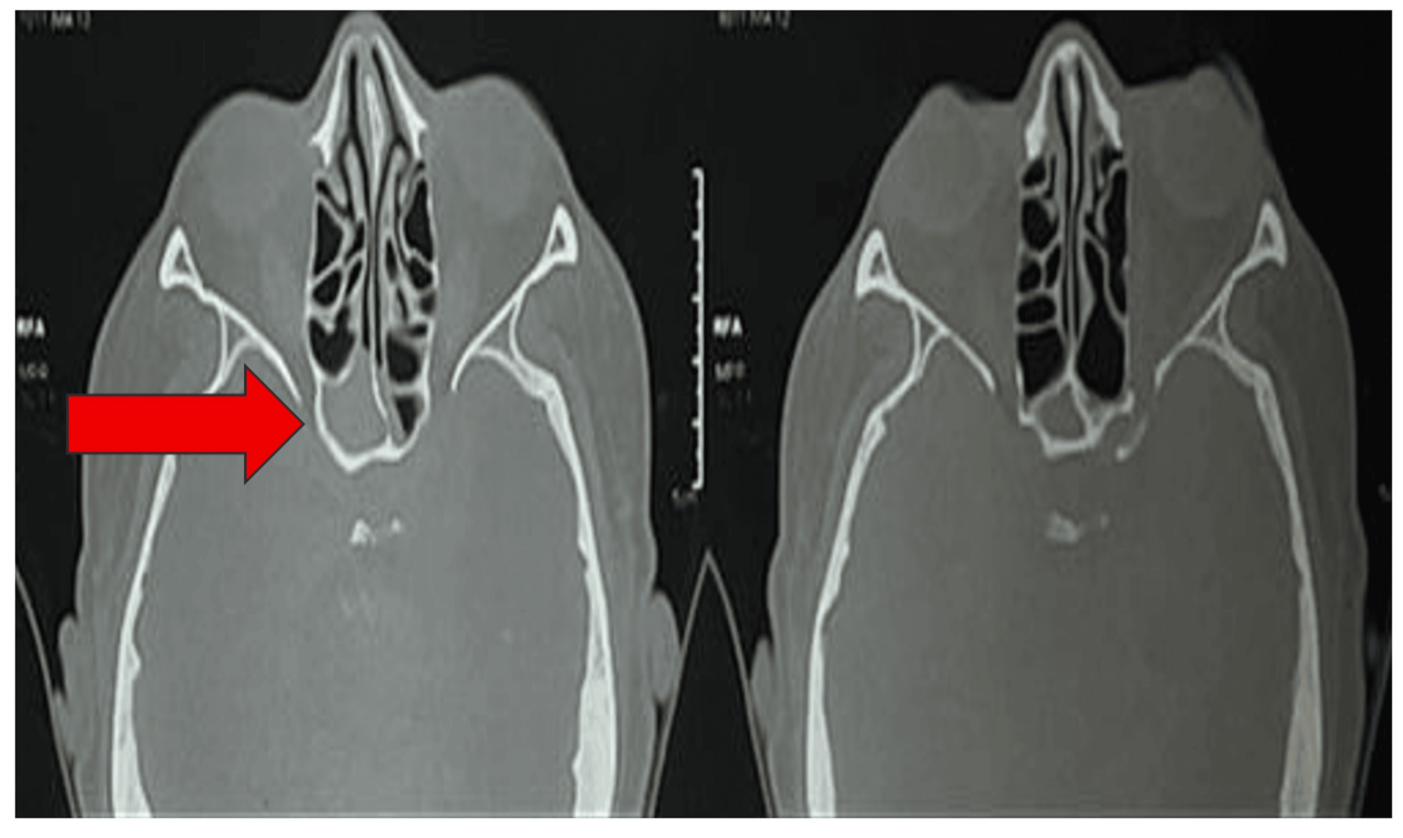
CT paranasal sinus of case 3. CT imaging demonstrated isolated mucosal disease (red arrow) in the right sphenoid sinus.
